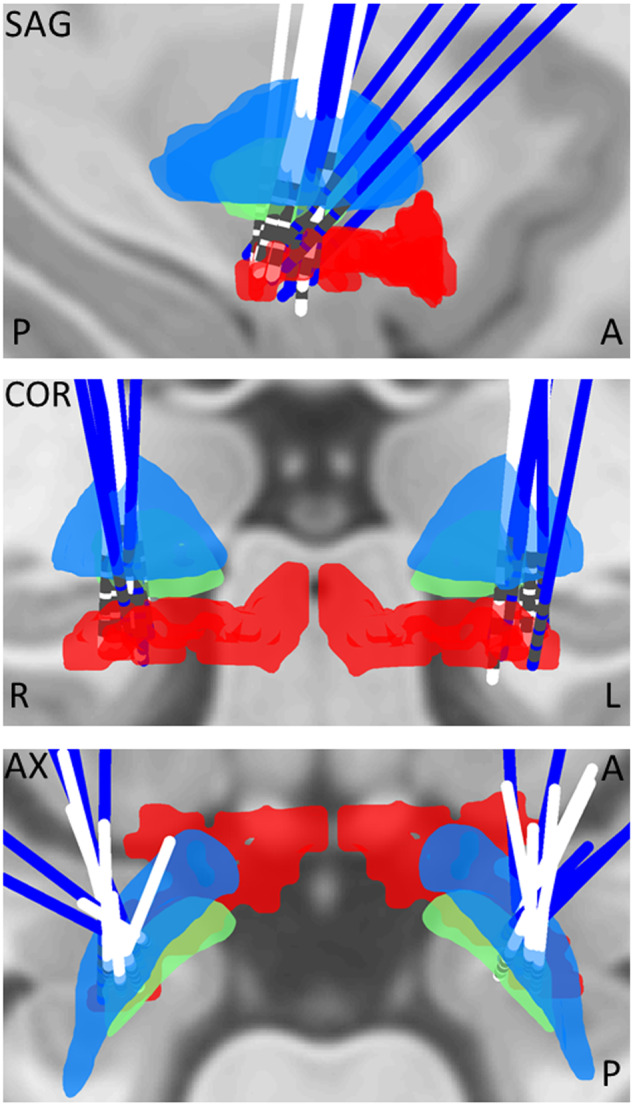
Localization of electrode trajectories and contacts in MNI space. Electrodes in the DLB cohort are coloured in dark blue whilst electrodes in the PDD cohort are coloured white. Templates of GPi (green), GPe (light blue) and basal forebrain (red), which includes the NBM are superimposed on a T_1_-weighted structural MRI for visualization. The 3D image is viewed in the axial, sagittal and coronal planes. A = anterior; Ax = axial; COR = coronal; L = left; P = posterior; R = right; SAG = sagittal.
